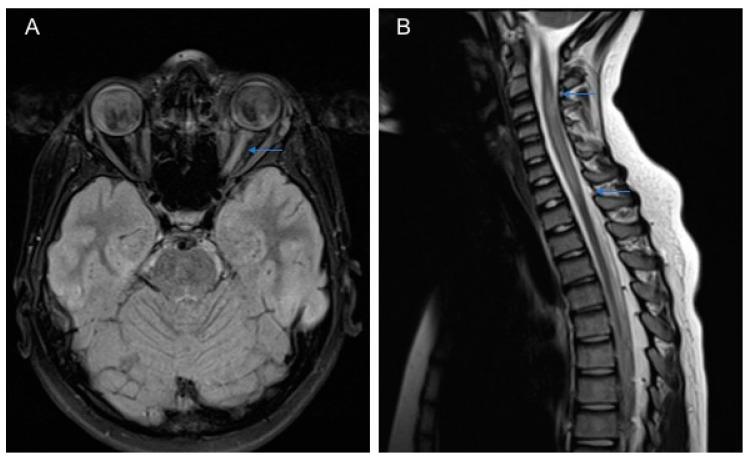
MRI of a 11-year-old patient with Neuromyelitis optica (NMO): (A) the brain axial Flair-T2 weighted image shows hyperintensity of the left optic nerve and (B) the spinal T2 weighted image shows cervical hyperintense lesions extending longitudinally from C3 to C7 (longitudinally extensive transverse myelitis (LETM).
